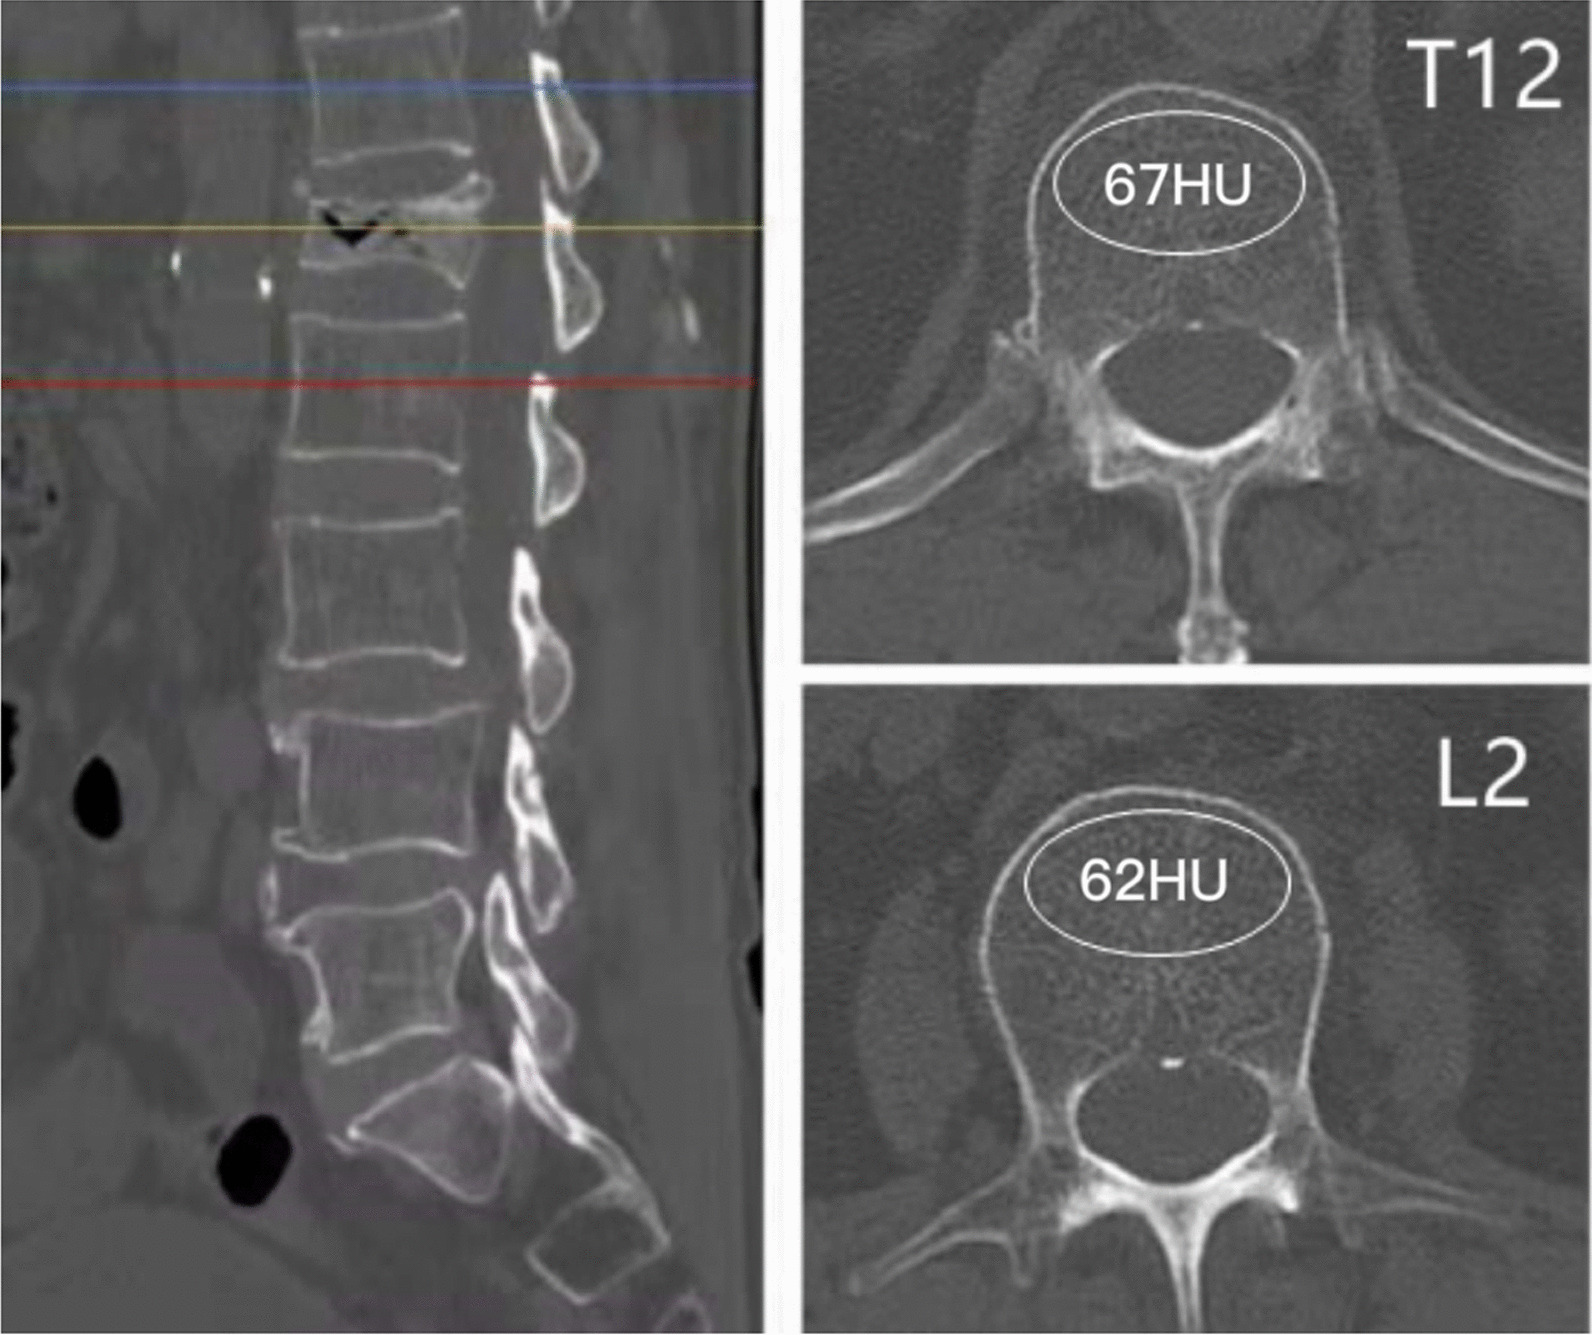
An 81-year-old male with a compression fracture of the L1 vertebral body. The average cancellous bone CT HU value of the T12 and L2 vertebral bodies was determined because of severe compression
